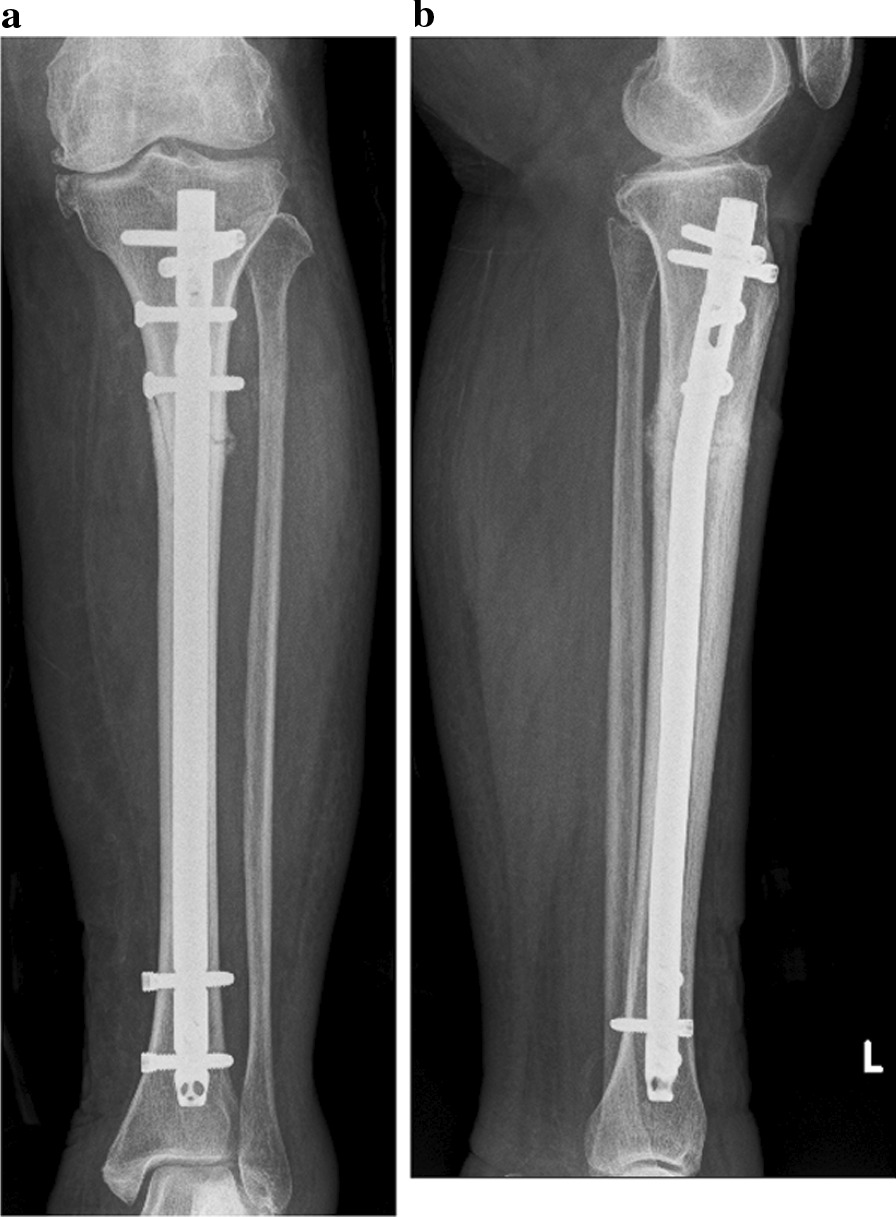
Two-directional X-ray image of her lower leg just after surgery. The intramedullary nail used was the Tibial Nail Advanced (φ10.0 mm, length 270 mm, DePuy Synthes, Paoli, PA)
